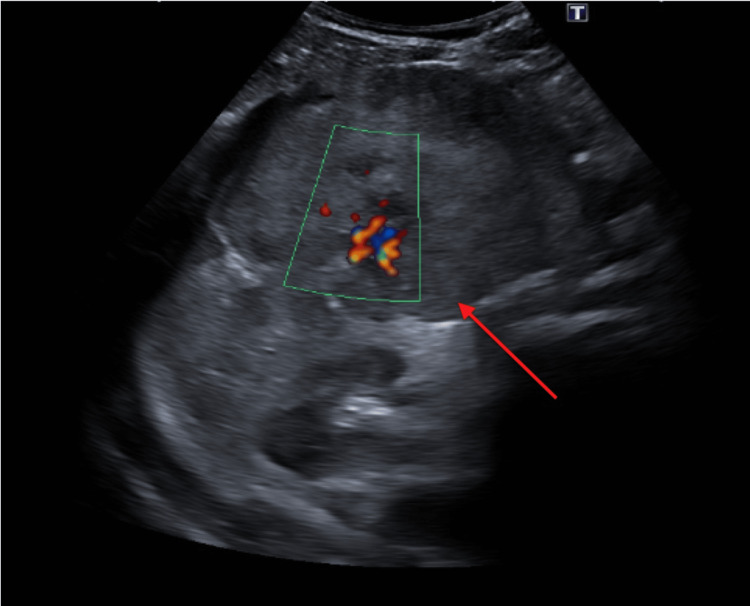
Abdominal ultrasonography showing a solid nodular formation measuring 113 x 94 mm with a color Doppler signal involving the left lobe and part of the right hepatic lobe.
